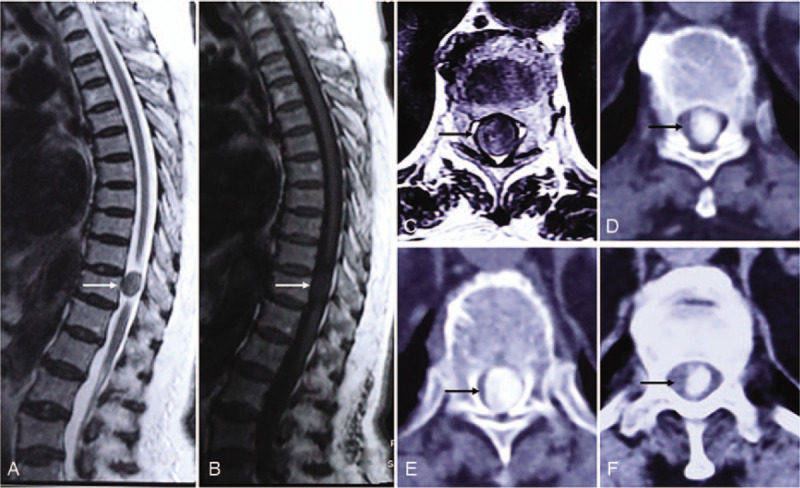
Magnetic resonance imaging revealed a mass at the T11 level. Compared to the spinal cord, the mass presented as an isointense signal on T2- and T1-weighted sequences (A and B). A computed tomography scan revealed a completely ossified mass in the spinal canal at the T11 level. The mass had clear borders and a high signal was evenly distributed within the entity (D, E, and F). The mass had a diameter that was almost equal to the inner diameter of the corresponding spinal canal, thereby causing serious compression to the nearby spinal cord (C, D, E, and F).
